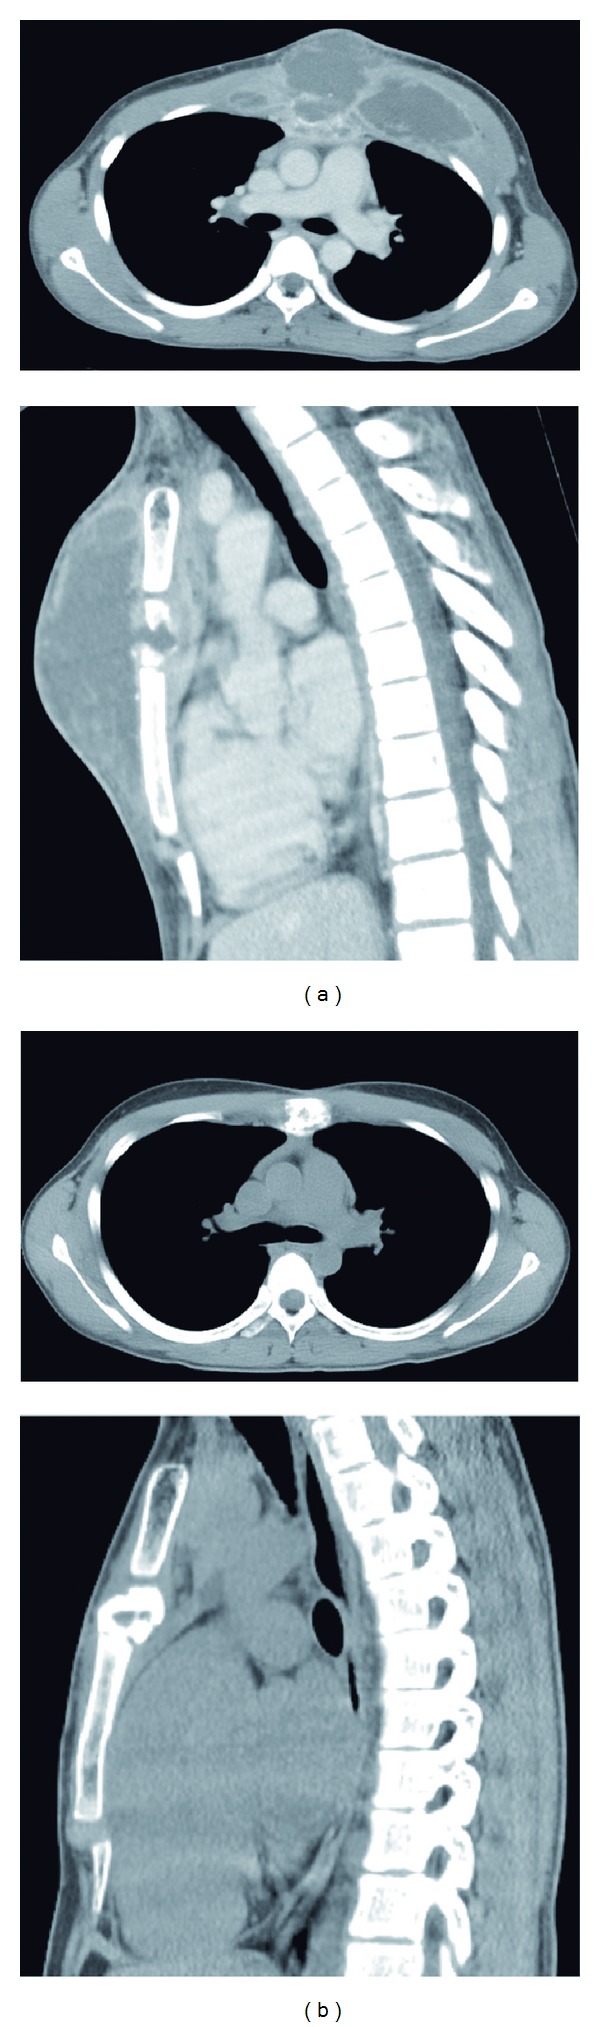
(a) Chest CT scan (upper) and reconstructed sagittal image (lower) revealed a sternal fracture and massive abscess formation. (b) Follow-up CT revealed the disappearance of fluid collection.
